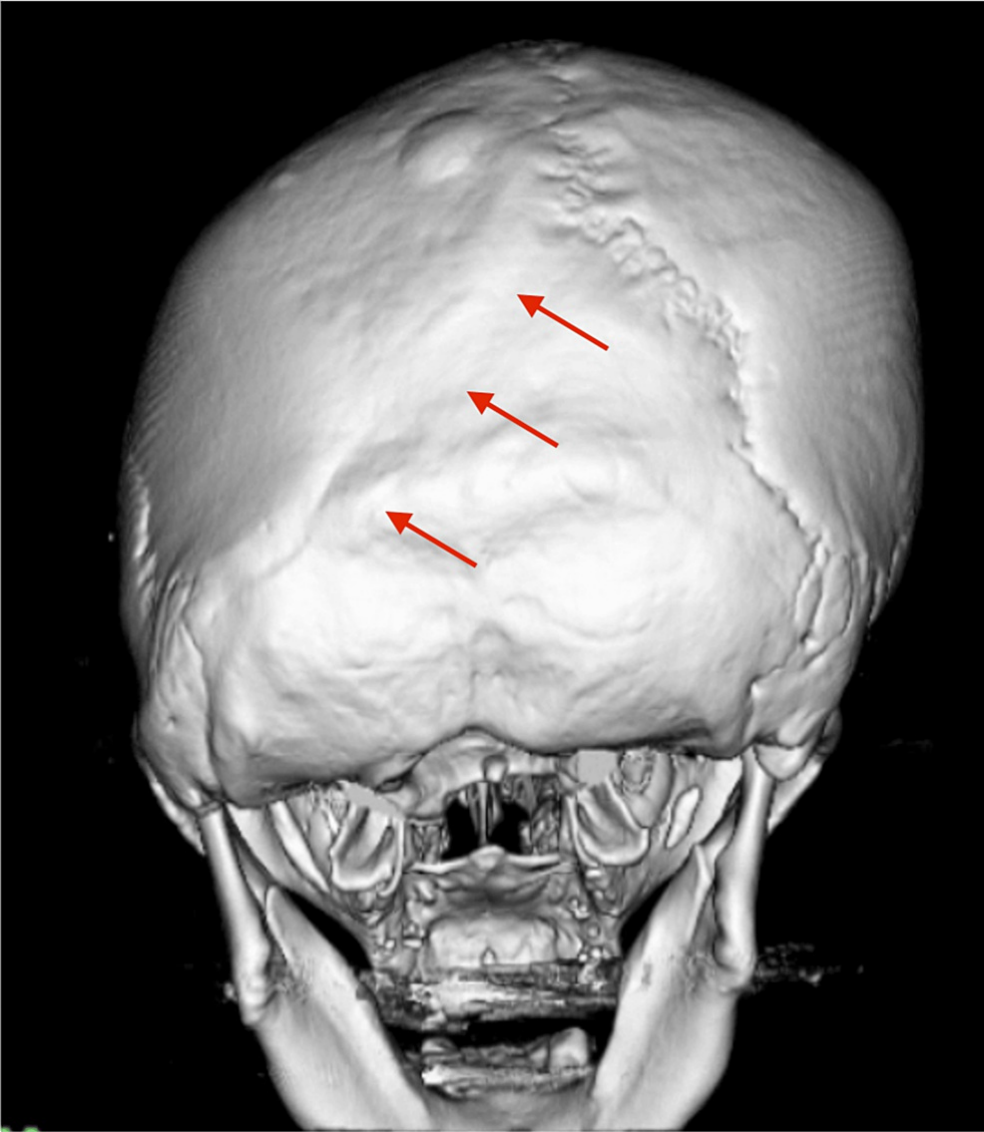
CT three-dimensional reconstruction. The CT scan shows a deformity of the head without clear identification of the left lambdoid suture. CT: computed tomography
